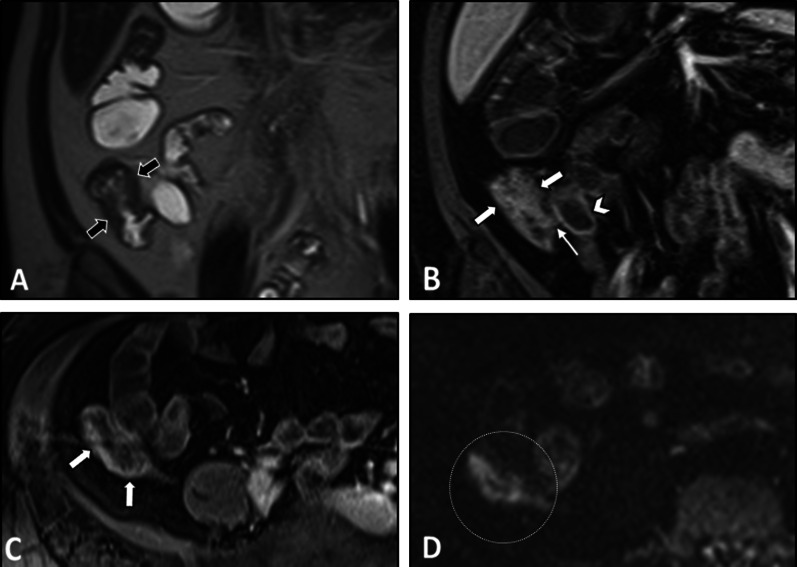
Intestinal vasculitis in a 26-year-old female with known Behçet’s disease and positive HLA-B5 complaining of episodic RLQ pain. MRE was obtained. Coronal T2-W image (A) demonstrates irregular circumferential mural thickening of the cecum and proximal ascending colon (thick black arrows) without mesenteric side predilection or pericolic infiltration. Coronal and axial post-contrast T1-W images (B, C) show marked enhancement of thickened colonic wall (thick white arrows) mildly extending to ileocecal valve (thin white arrow) with low-grade mural hyperenhancement in the adjacent terminal ileum (white arrowhead). Axial DWI image (D) obtained at a b-value of 800 s/mm^2^ reveals restricted diffusion in the thickened cecum (white dotted oval)
